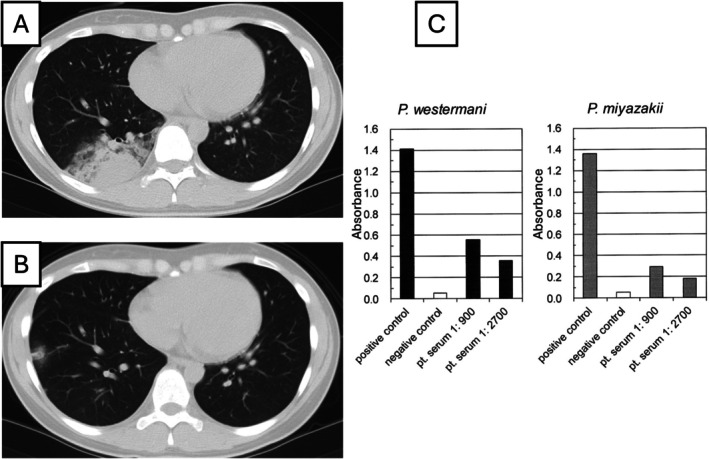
High‐resolution computed tomography (HRCT) images of Case 1 and results of antiparasitic antibody testing (microplate enzyme‐linked immunosorbent assay). (A) HRCT image obtained at the initial visit to the referring physician showing peripheral airspace consolidation in the right lower lobe. (B) At the time of initial presentation to our hospital, the consolidation showed partial resolution, whereas new nodules were observed in the right lower lobe. (C) Pretreatment serum antiparasitic antibody testing was positive for *Paragonimus westermani* and *P. skrjabini miyazakii*.
